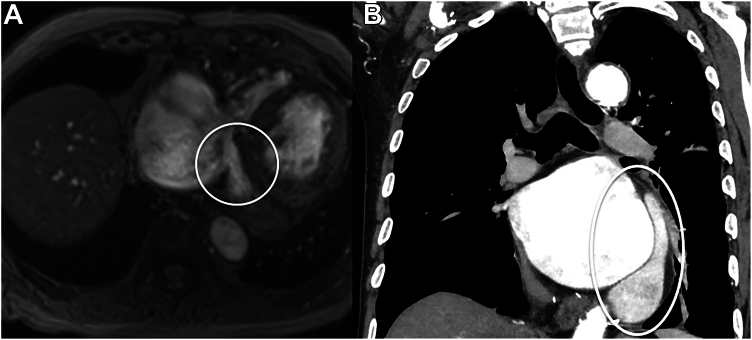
(A) Magnetic resonance imaging shows reopening of the coronary sinus ostium with direct right atrial drainage (circle). (B) Coronal computed tomography shows improved coronary venous washout (oval).
